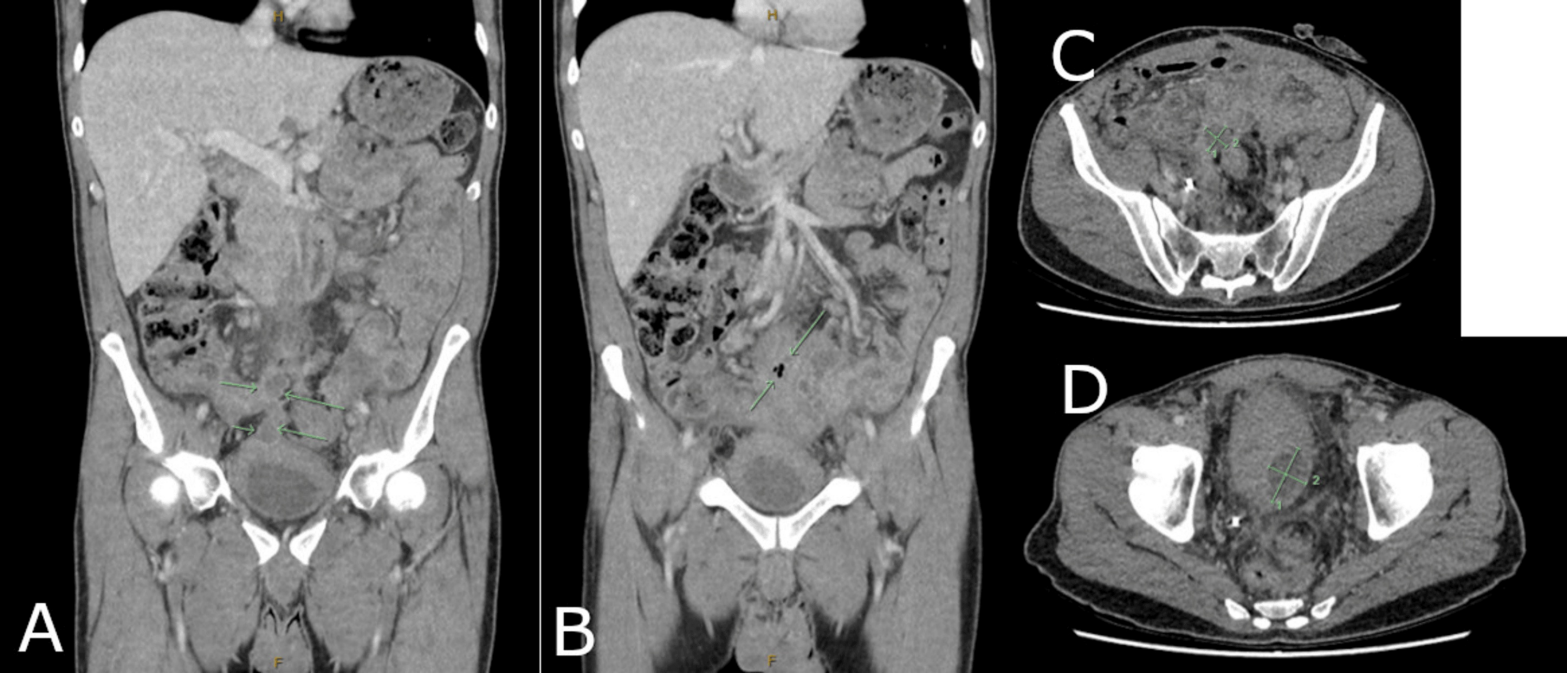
CT images demonstrating pelvic abscess on coronal slices (A,B), and axial slices (C,D)
